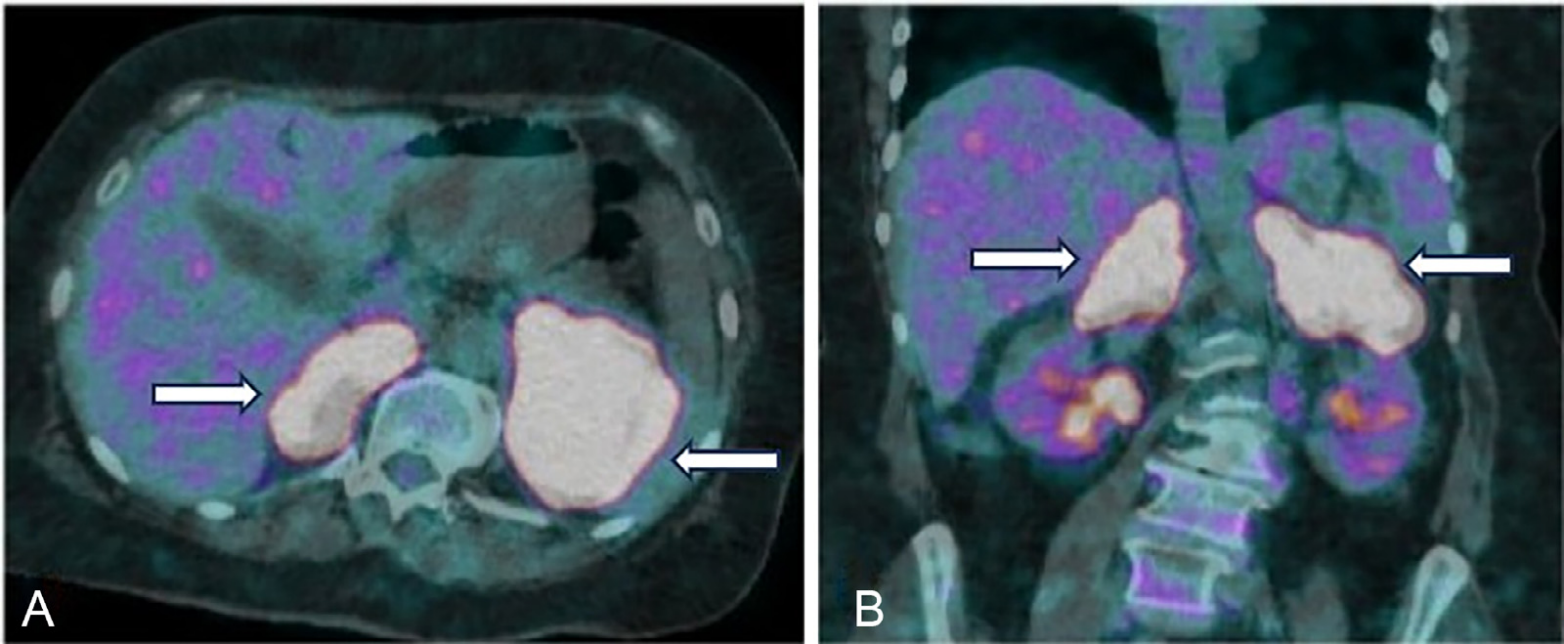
Axial and coronal fusion images from baseline PET-CT at diagnosis, showing hypermetabolic bilateral adrenal masses denoted by arrows, consistent with biopsy-proven lymphoma.
